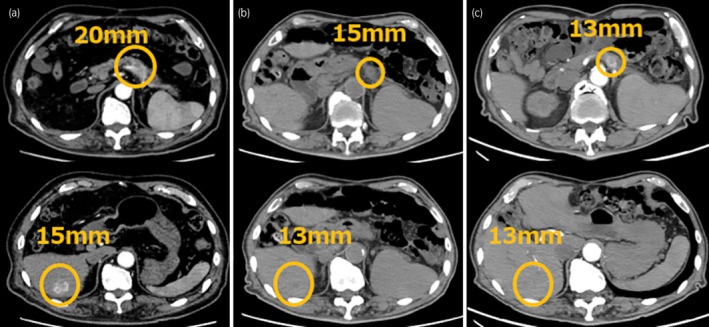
Computed tomography of pancreatic and liver metastases before administration (a), interruption (b), and resumption (c) of ICI.
